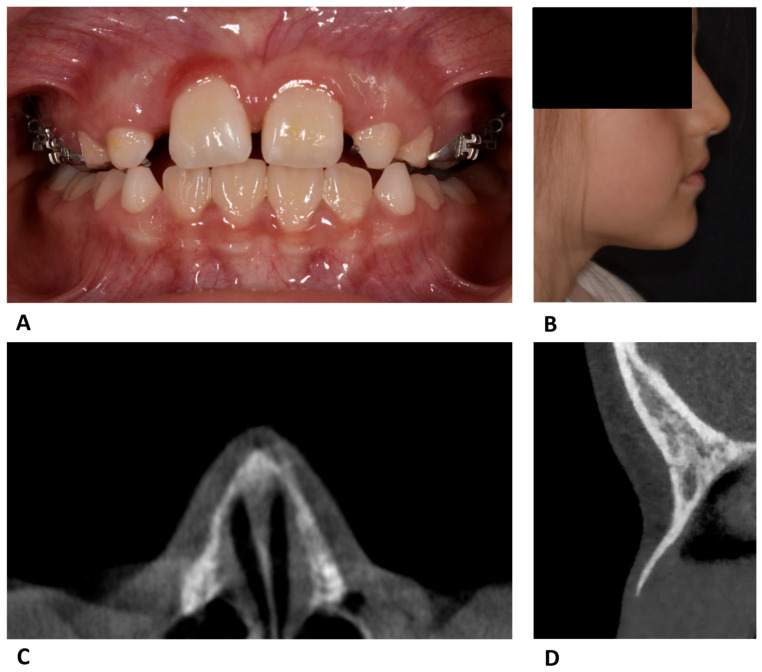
(A) Intraoral photograph at the follow-up visit. (B) Extraoral profile photograph. (C) CBCT axial slice showing bilateral continuity. (D) CBCT sagittal slice showing continuity of the nasal bone.
